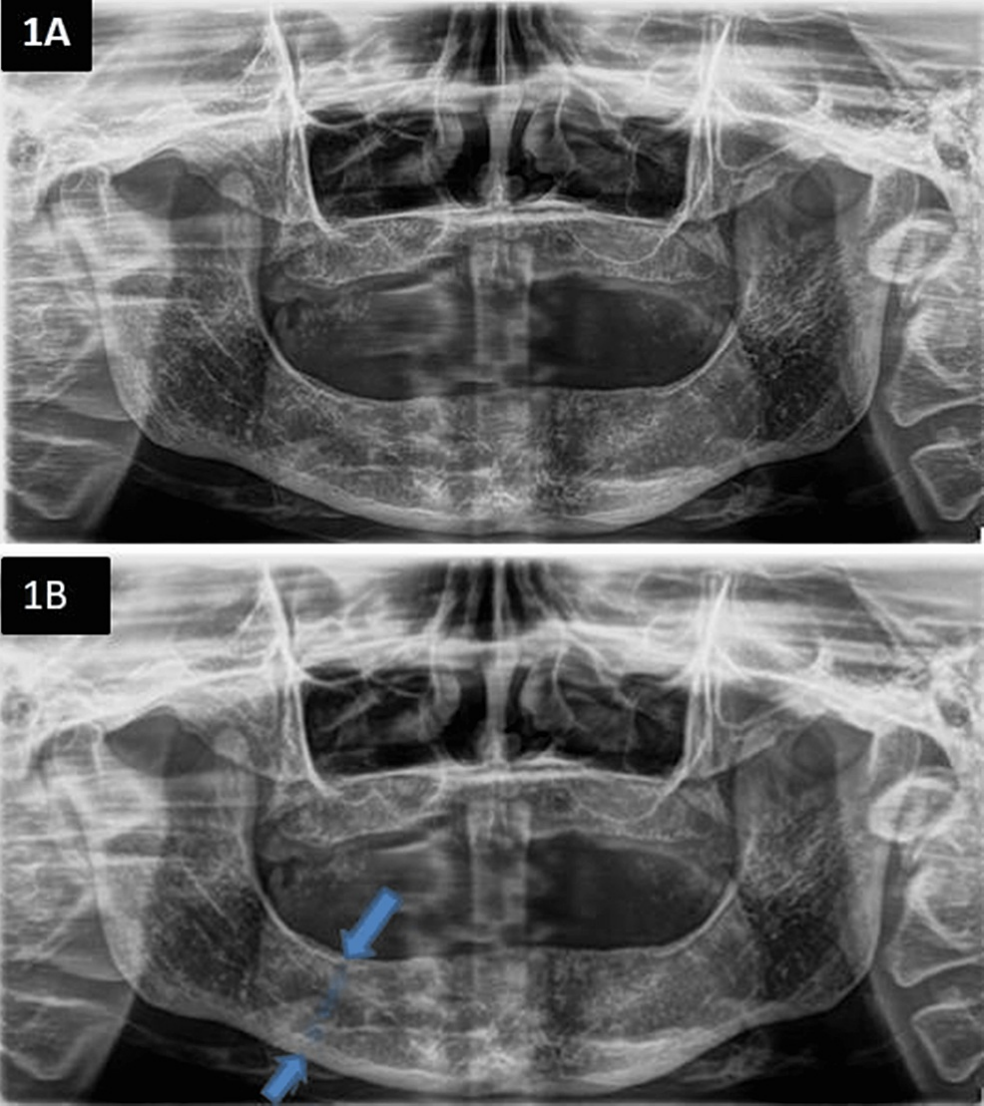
(A-B) Pre-operative orthopantomogram of the patient, with the bone height measured (arrows) using CorelDRAW Graphics Suite X6
